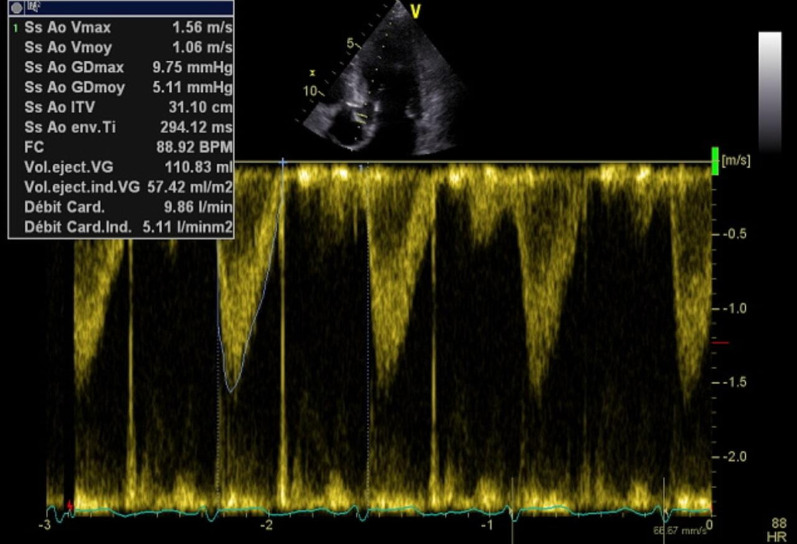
Transthoracic echocardiogram image: cardiac output measured at 9.8 L/min in two-dimensional imaging (stroke volume multiplied by heart rate)
